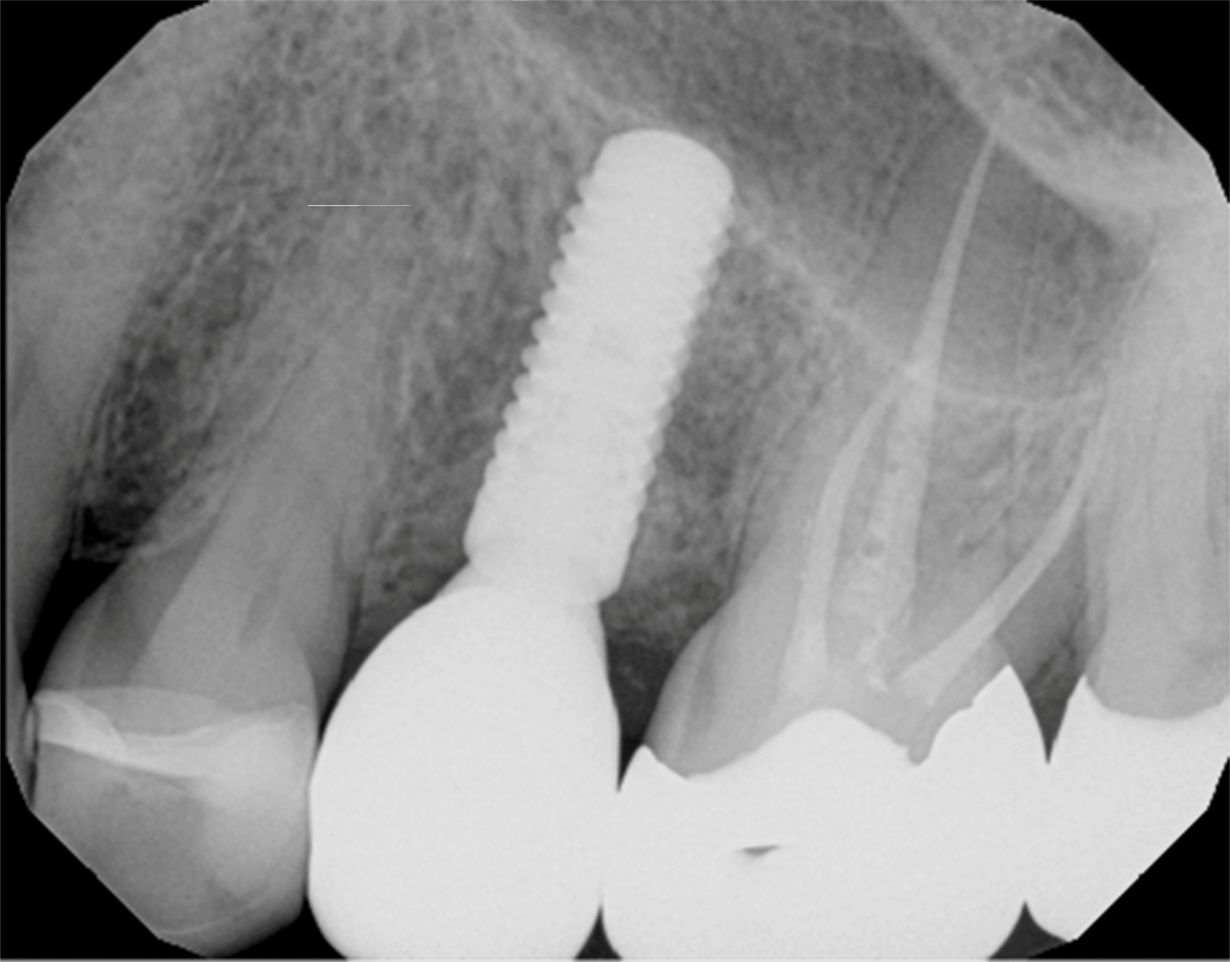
Radiograph of the implant at #13 demonstrating good bone levels and no noticeable issues
